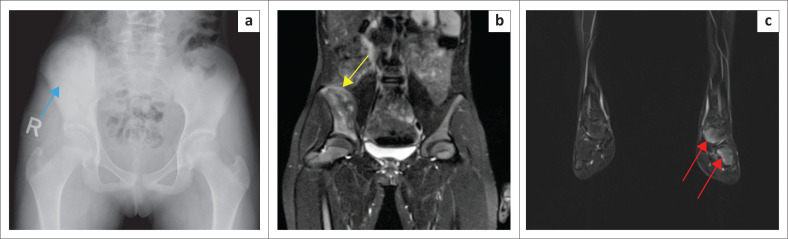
Follow-up imaging with a radiograph of the pelvis and whole-body magnetic resonance imaging (MRI) performed 3 months after treatment: (a) Plain radiograph of the pelvis showing similar findings of sclerosis of right iliac wing. (b) Magnified fat-saturated T2 weighted coronal image on whole-body MRI shows significant improvement of the oedema and surrounding periosteal reaction (arrow). Note the absence of adjacent muscle inflammation. (c) Magnified section of the whole-body MRI, coronal T2 weighted fat-saturated MR image of the left lower leg showing increased marrow signal involving the inferior talus, cuboid (arrows) and base of 5th metatarsal bone (not shown). This involvement of multiple bones with regression of inflammation in the ilium after conservative treatment confirms the diagnosis of chronic recurrent multifocal osteomyelitis.
